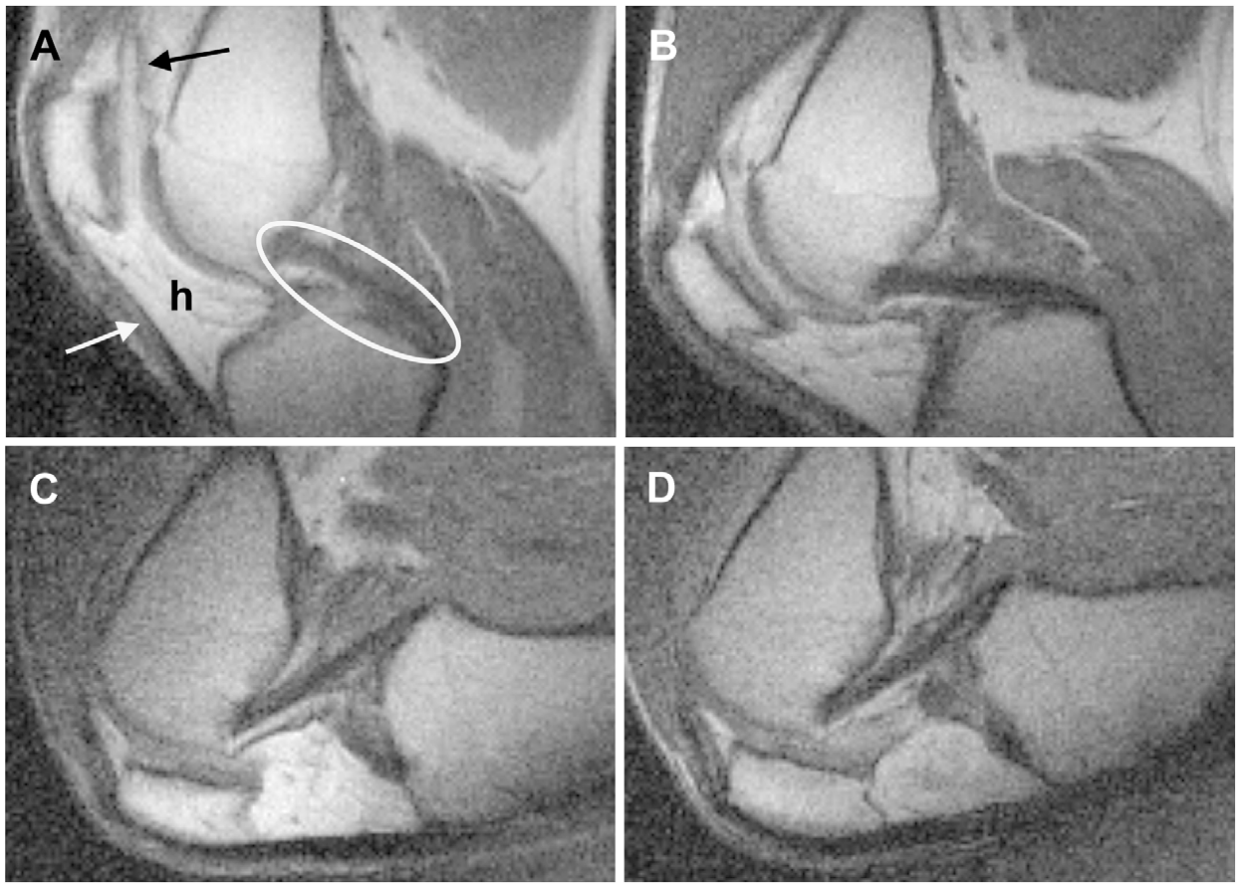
Four of the 2D MR images that were stitched together to make the 2D movie. The anterior of the knee is to the left, the posterior is to the right. Knee flexion angles were approximately 35° (A), 65° (B), 105° (C) and 120° (D). Soft tissues visible include the PCL (oval), patellar tendon (white arrow), Hoffa’s fat pad (h), and synovium (black arrow).
